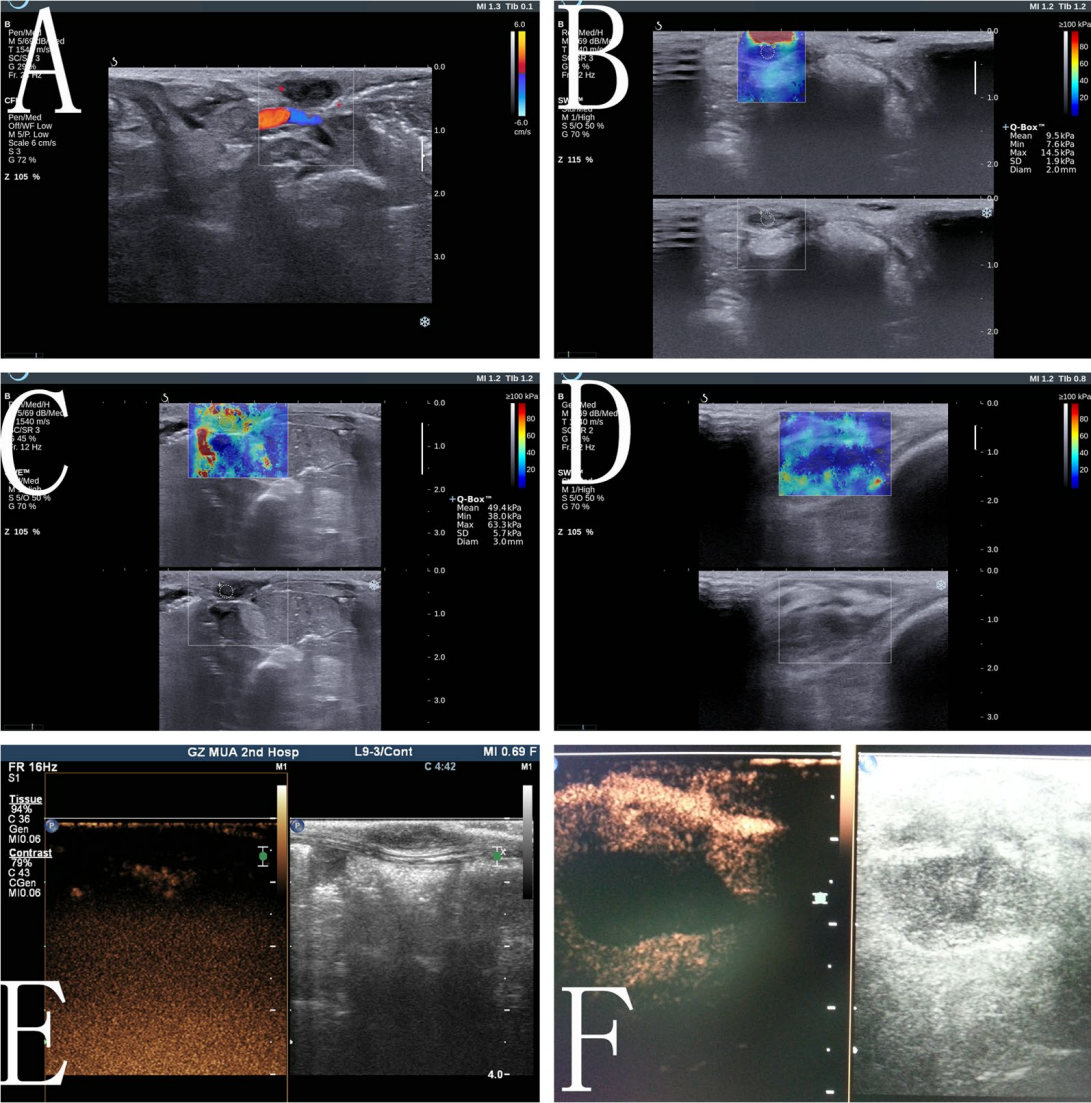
Contrast-enhanced ultrasound and elastosonography before and after IRE ablation of breast cancer in a rabbit model. a, b Ultrasound and elastosonography before IRE showed the echo and rigidity of the ablation zone. c, d After IRE, elastosonography led to an increase in the rigidity value (1 day after IRE), followed by a decrease (7 days after IRE). e Based on contrast-enhanced ultrasound after IRE, there was no enhancement in the ablation zone (1 day after). f Based on contrast-enhanced ultrasound, 7 days after IRE, the area without blood supply had enlarged
